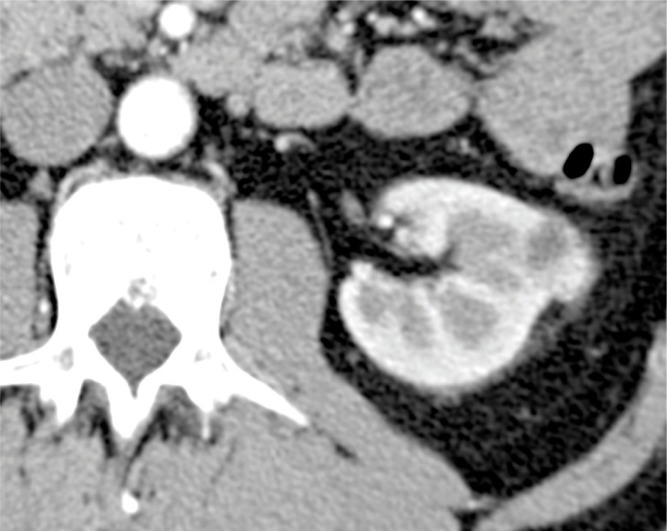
CT axial scan during arterial phase of a 44-year-old male (same patient of Figure 3) shows a minute hypervascular solid component located in the cranial portion of the clear cell renal cell carcinoma.
